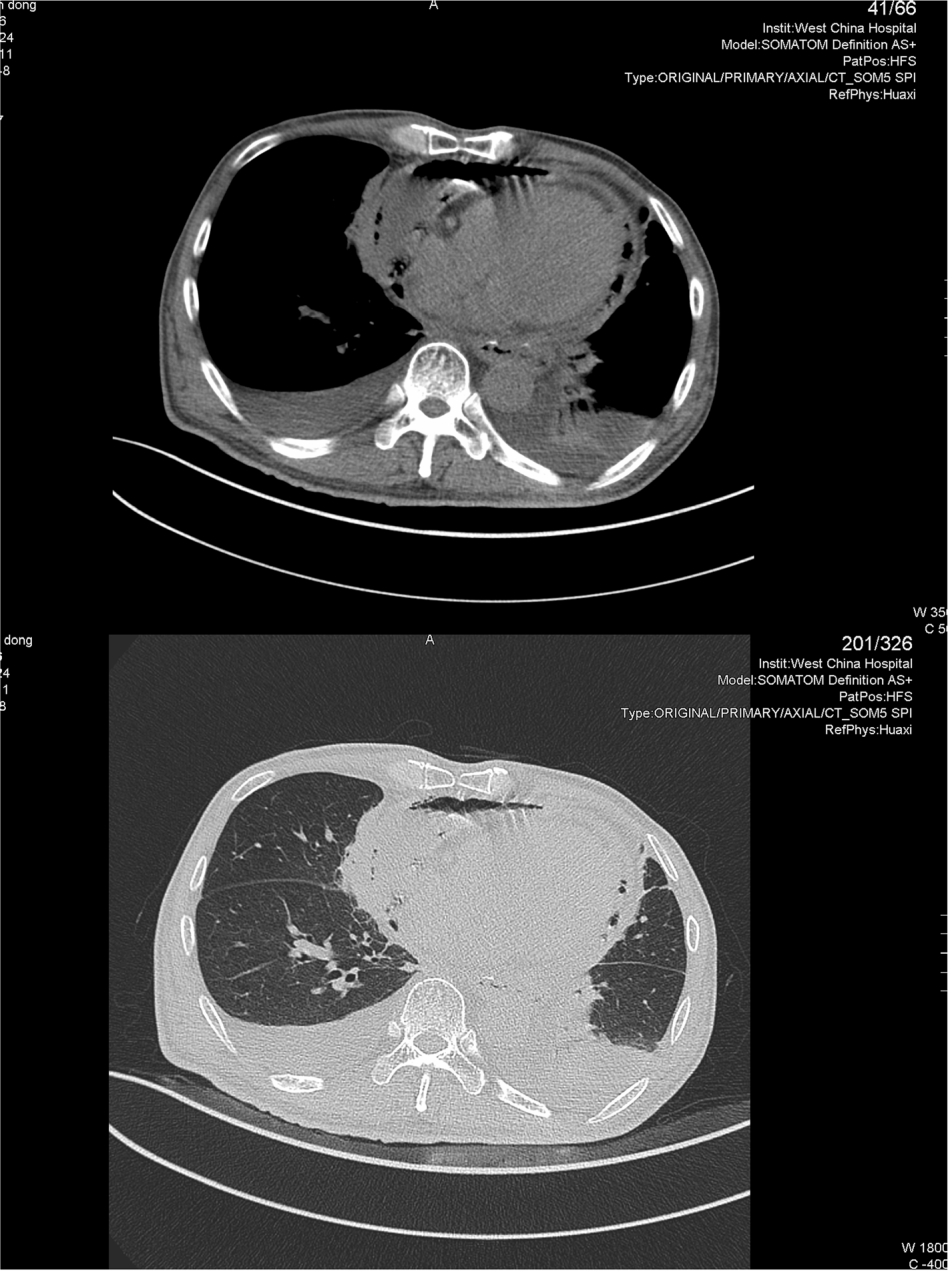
CT scan of the thorax at mid-thoracic level showing widened mediastinum, pyopneumopericardium, bilateral pleural effusion, and collapse of the lower lobe of left lung
